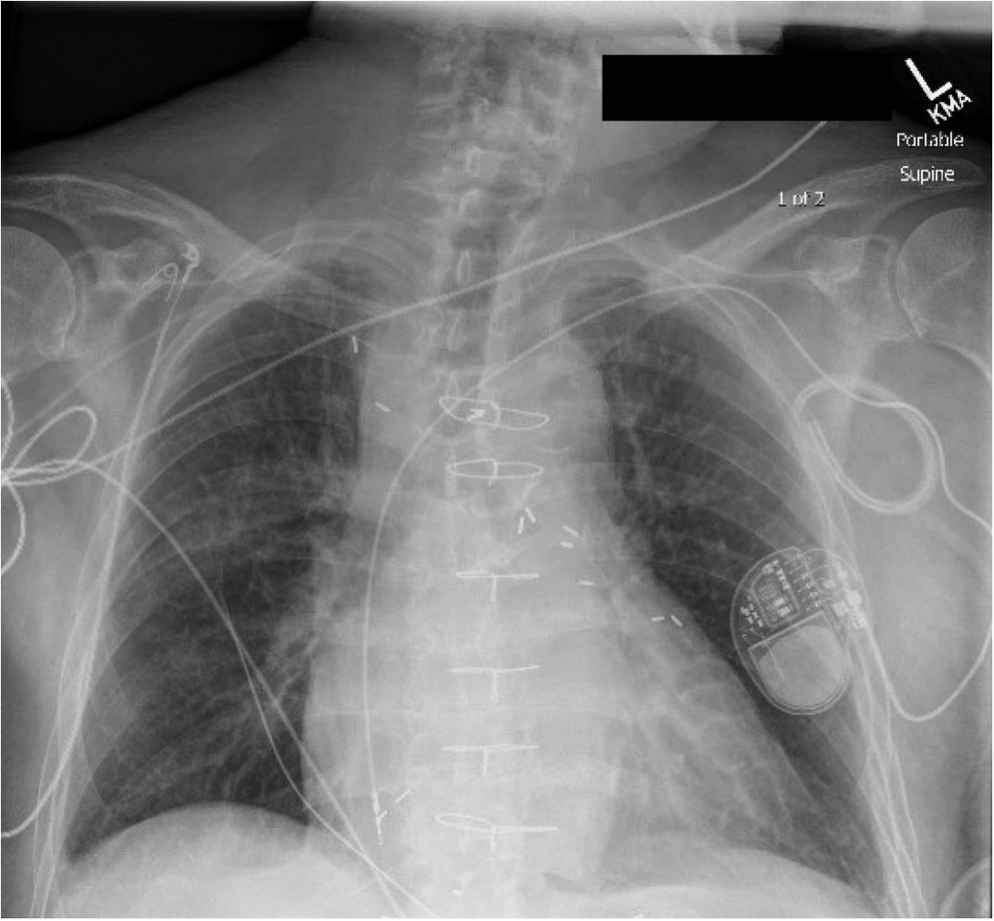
Postprocedural chest radiograph revealing removal of all biventricular implantable cardioverter-defibrillator leads, insertion of temporary wire to the right ventricular apex, and noncentral peripherally inserted vascular access device.
